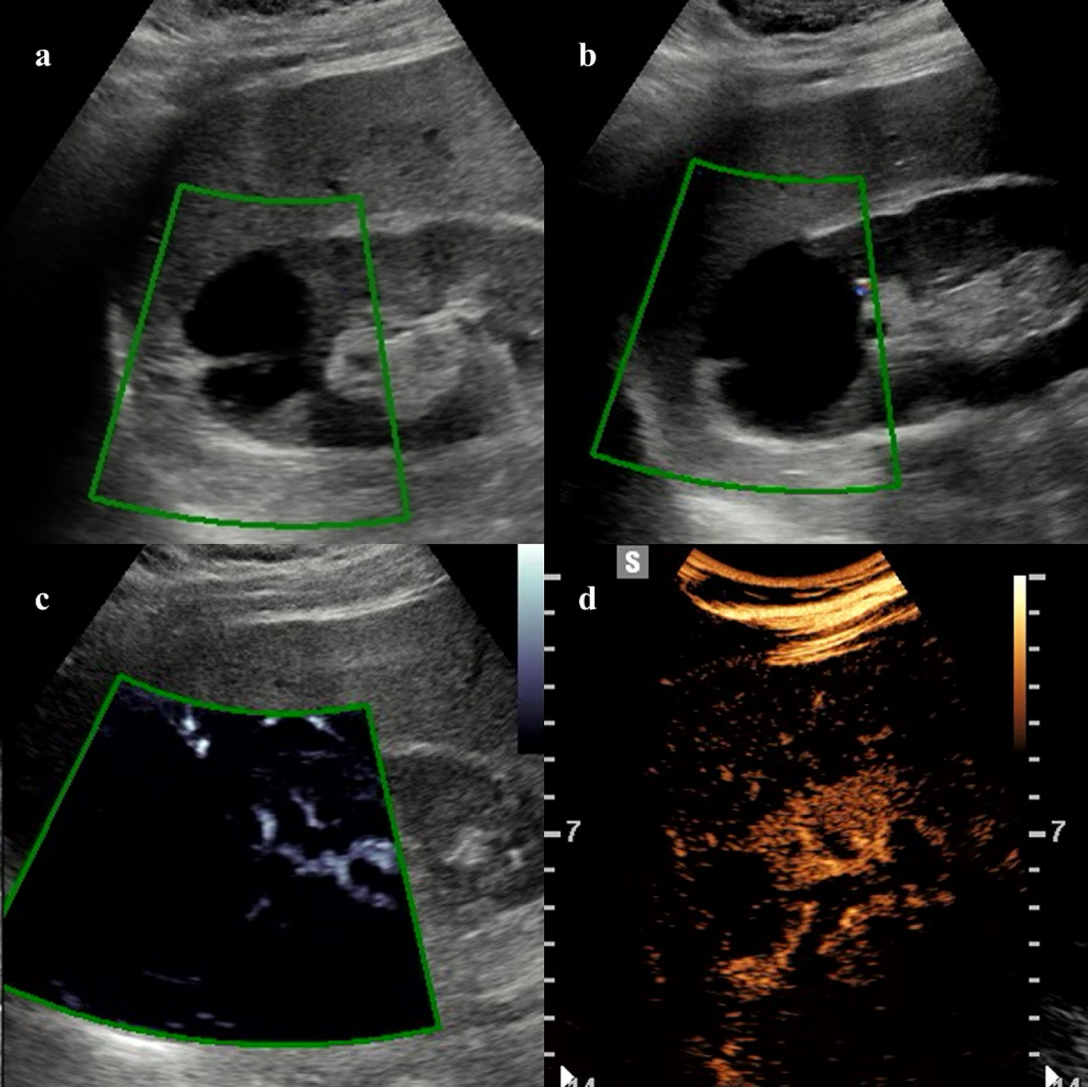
56-year-old man with 5 cm cystic renal lesion detected on computed tomography. Color (a), directional power doppler (b), and microvascular flow imaging (c) do not detect any intracystic vascularization. Contrast-enhanced ultrasound (d) shows septa with minimal enhancement at 58 s after contrast injection. The lesion was classified as bosniak IIF
